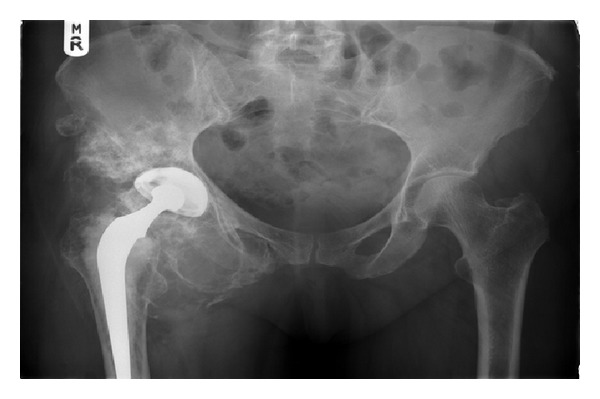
Plain X-ray of case 4 preoperatively.
